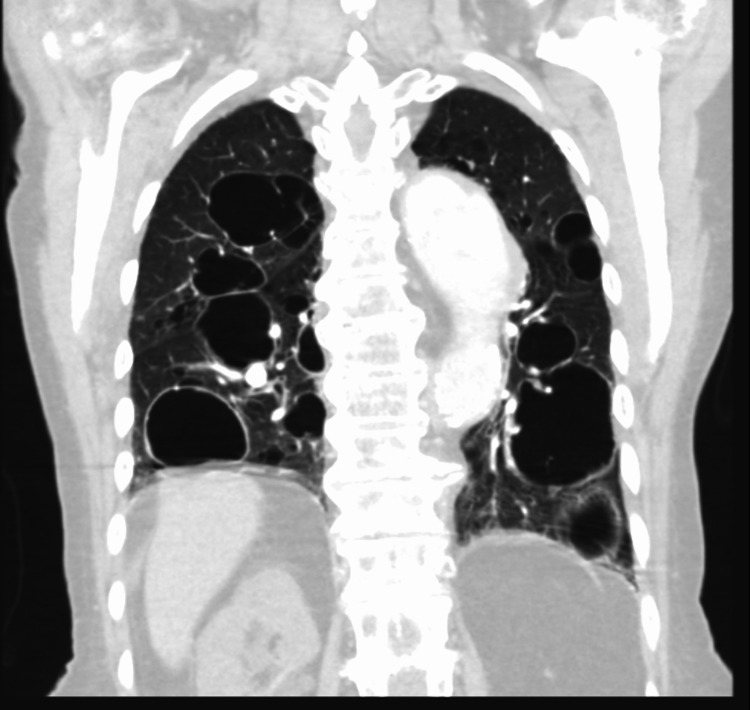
Coronal view of the chest CT demonstrating bilateral cysts
